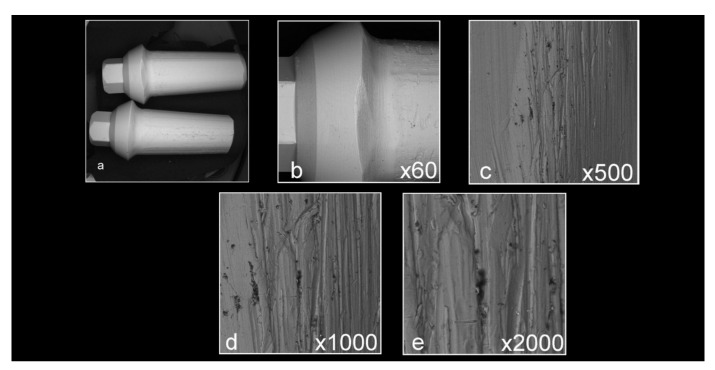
Abutments that were prepared in a lab and decontaminated using ultrasonic washing ((a) no magnification; (b) ×60 magnification; (c) ×500 magnification; (d) ×1000 magnification; (e) ×2000 magnification). The samples have some debris and accumulation of contaminants, but look smoother compared to the control samples shown in Figure 2.
